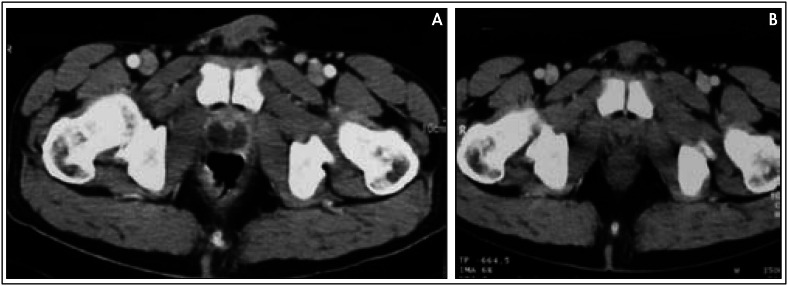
A. Computed tomography examination showing a large prostatic abscess with necrosis. B. Computed tomography examination after forty days of treatment, showing a normal size prostate gland with a minimal necrotic abscess.
